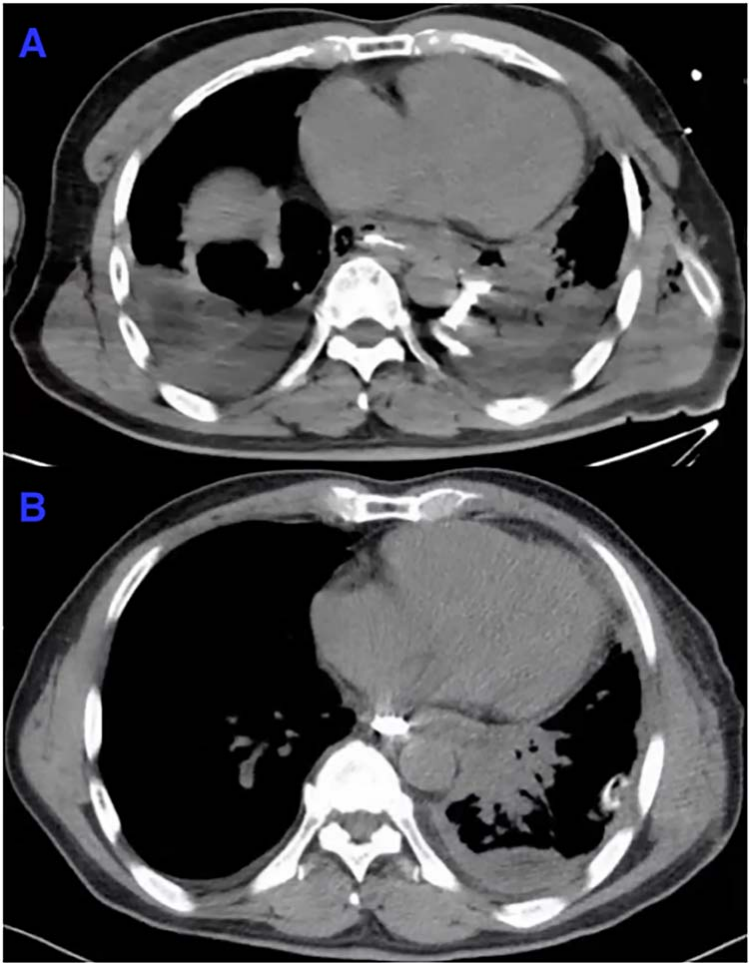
CT oesophagography. Axial slice. (A) Horizontal leakage of oral contrast is shown from distal oesophagus to the mediastinum. CT also revealed bilateral pleural effusion on admission. (B): On POD10, repeat CT oesophagography did not show leakage of oral contrast. Bilateral pleural effusion, as compared to A, has largely resolved.
